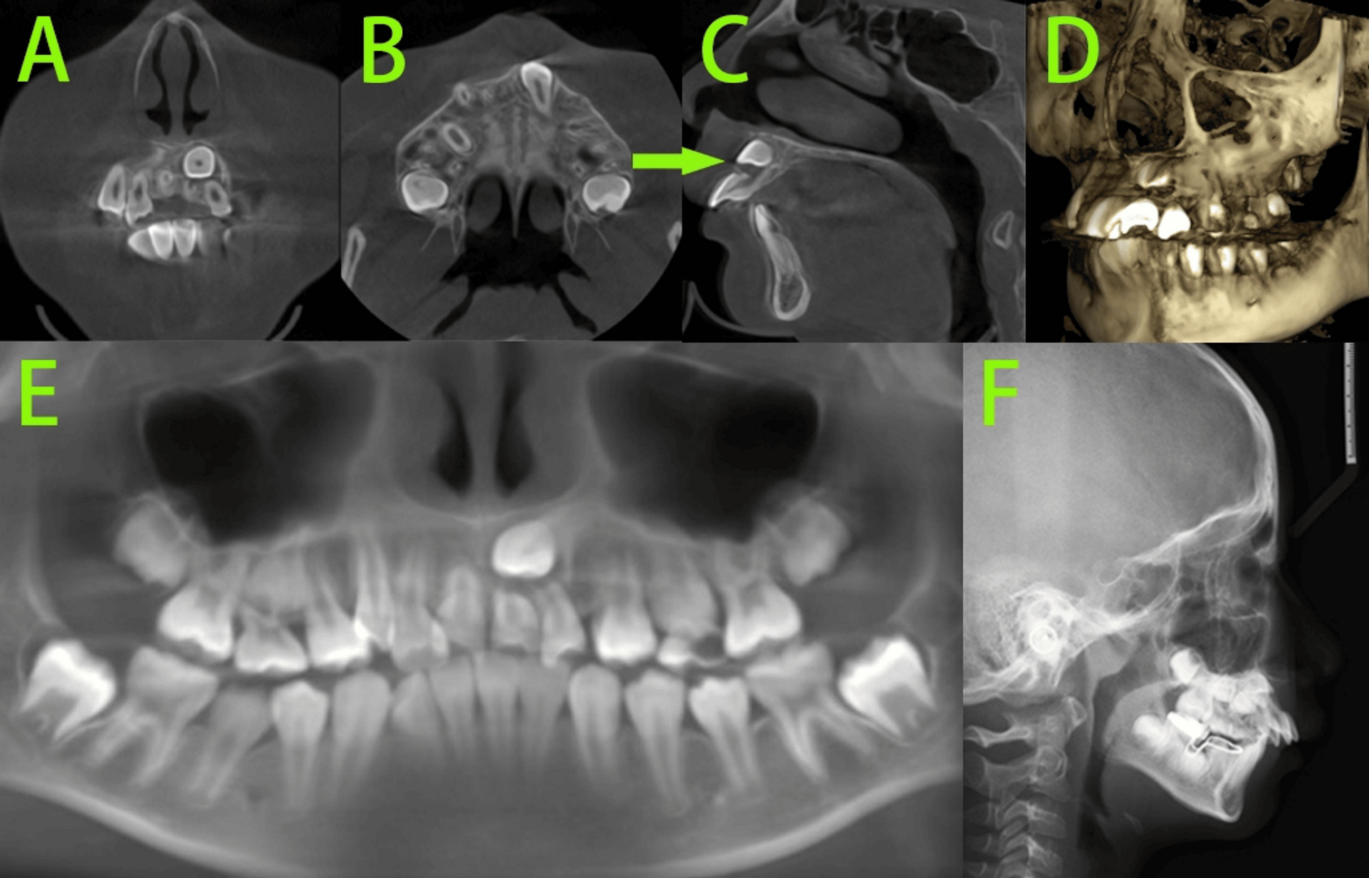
Pre-treatment radiographs A-C: CBCT revealed root resorption was observed in the retained primary teeth 55 and 65, tooth 23 was ectopic and impacted in the apical region of tooth 21, and significant root resorption of tooth 21 was already evident (green arrow). D: CBCT 3D reconstruction reveals that tooth 23 is impacted on the labial side of the root of tooth 21. E: The CBCT-synthesized panoramic image showed mesial inclination of the axis of tooth 23. F: lateral cephalometric radiograph taken at another hospital six months ago indicated that the patient was in the pre-peak growth period, a skeletal Class II pattern with mandibular retrusion, a normodivergent facial angle, an average growth pattern, proclination of the maxillary incisors, and upright mandibular incisors.
